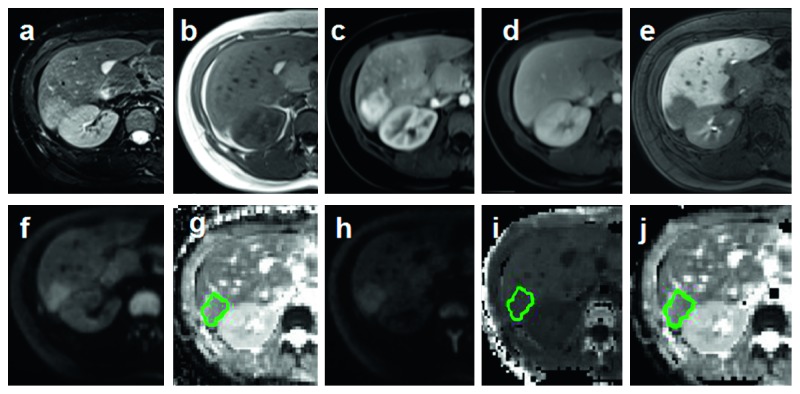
ROI was drawn on the level of maximum transactional diameter of HCA (green circle). (a, b) Axial fat suppress T2WI and T1WI. (c–e) Dynamic enhancement imaging included artery phase, portal phase, and hepatobiliary phase (HBP). (f, g) Axial liver DWI (*b* = 800 s/mm^2^) and ADC maps. (h–j) Axial liver DKI (*b* = 2000 s/mm^2^) and DKI-derived parameters *K* and *D* maps.
